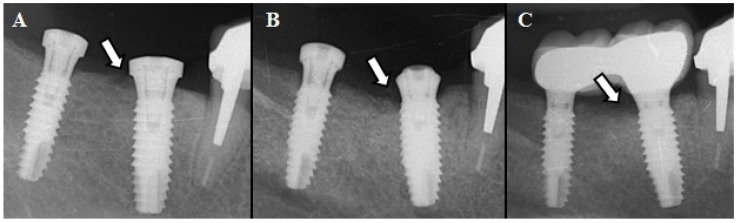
Radiographic control. A) Radiolucent image distal of 4.5 at 2 weeks of surgery (arrow); B) Bone resorption distal of 4.5 at 7 months (arrow); C) Maintenance of bone level at 1 year of prosthetic restoration (arrow).
